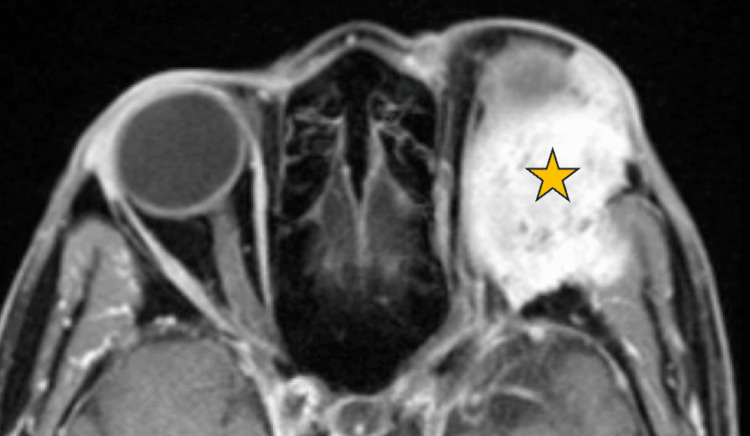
Axial T1-weighted magnetic resonance image (MRI) with contrast of bilateral orbits. The MRI demonstrates a large, destructive left spheno-orbital mass (gold star) extending into the anterior cranial fossa. The lesion produces marked proptosis and displacement of the left globe.
